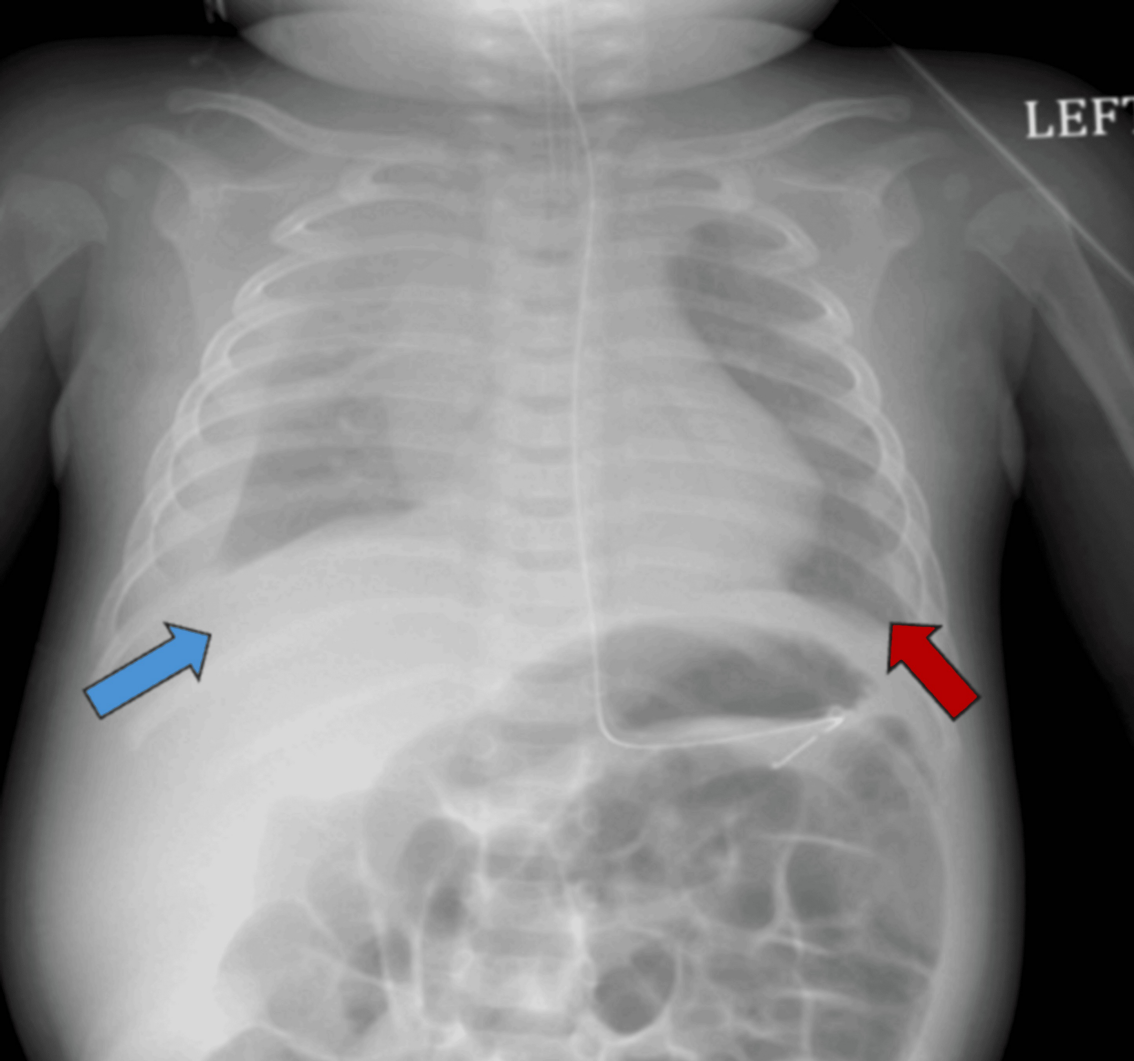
Frontal chest radiograph demonstrating a moderate-to-large right pleural effusion (blue arrow), with interval improvement compared to the prior study. A small, stable left pleural effusion is also present (red arrow). Diffuse hazy opacities are noted throughout the bilateral lung fields, without significant interval change.
